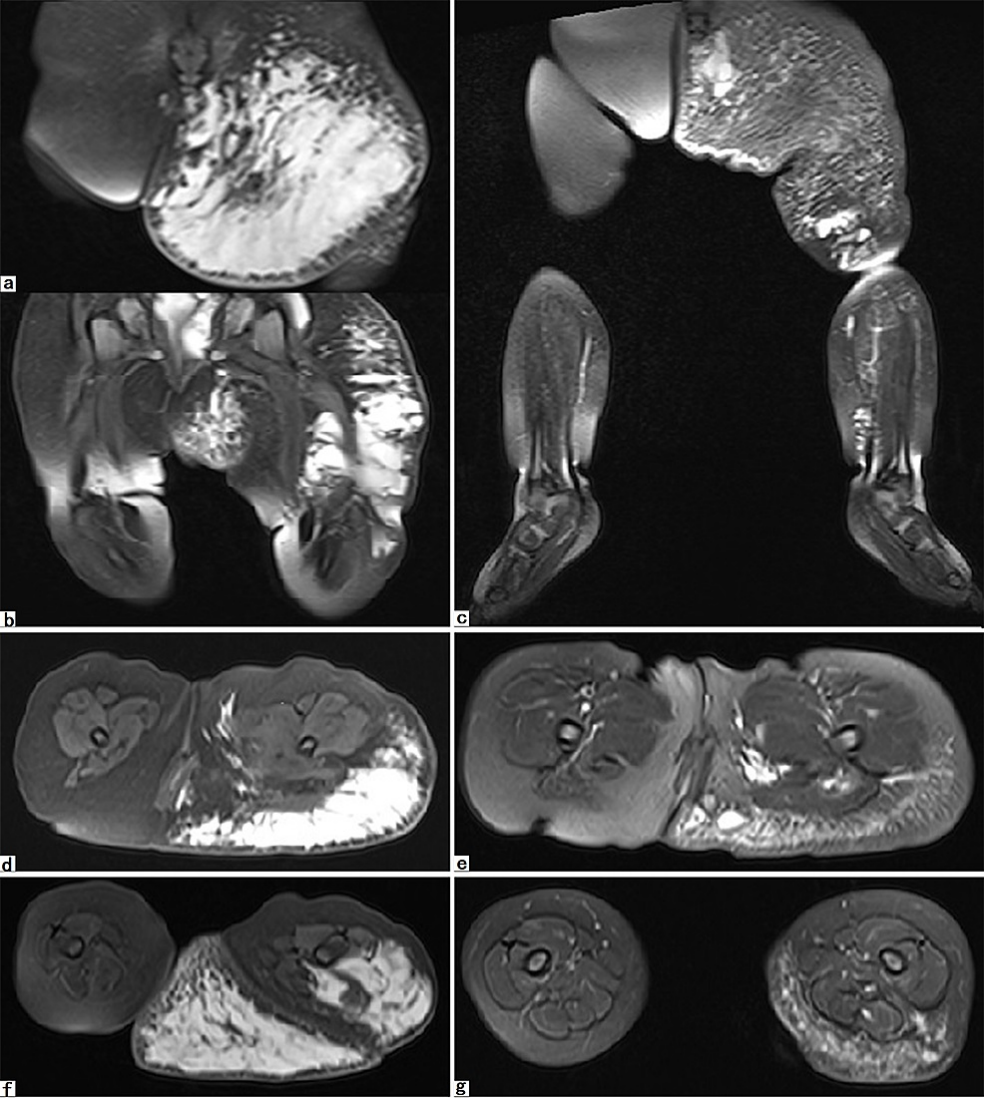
MRI of an extensive lymphatic malformation before and after treatment. Panel a, b, d, and f: An extensive mixed cystic lymphatic malformation with predominantly microcystic component involved entire left lower extremity and buttock. Panel c, e, and g: After 6 months following treatment, the lesion had largely decreased by imaging criteria. It was noticed that the obviously enlarged left buttock had a great improvement on MRI. Furthermore, a dramatic improvement in the intramuscular component was observed simultaneously, which implies interconnection with subcutaneous component. The result of treatment in this patient was graded as partial response.
